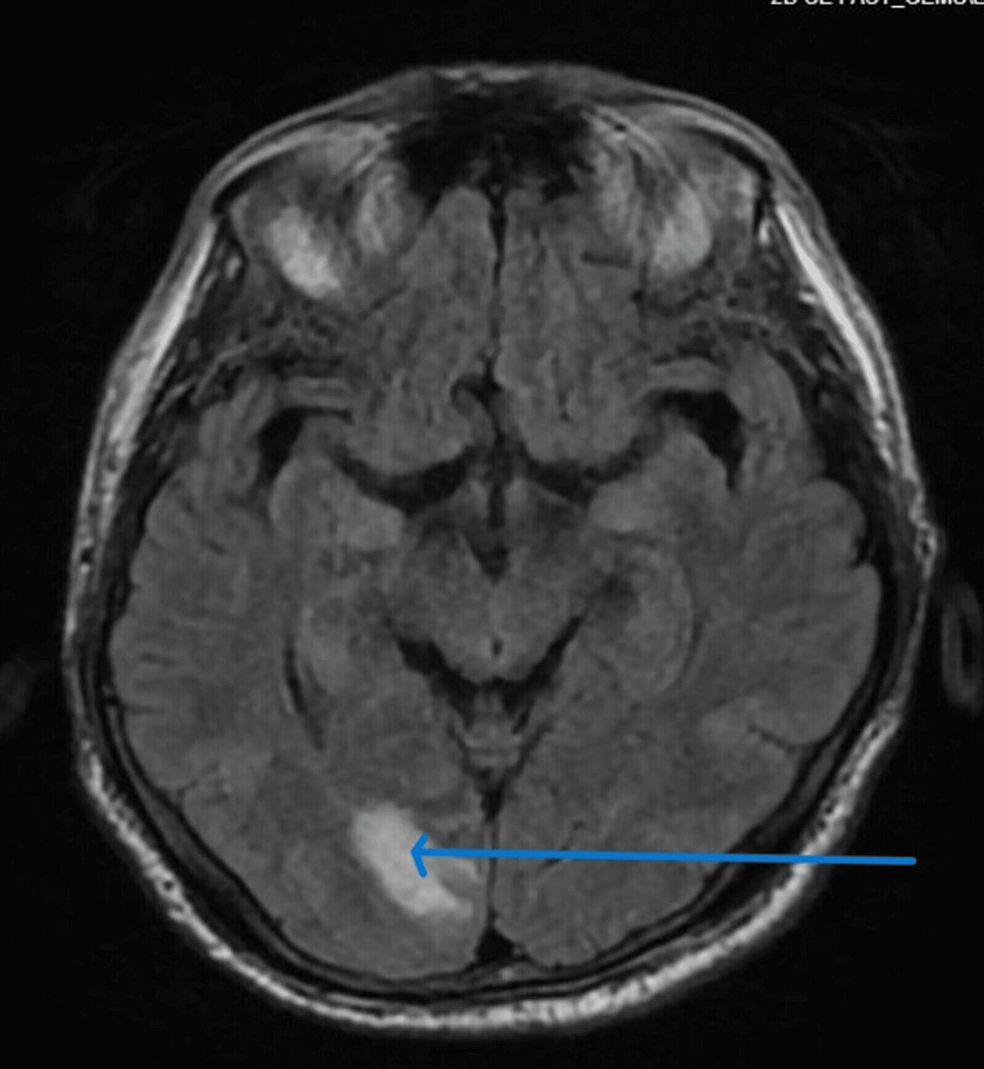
MRI of the brain demonstrating right occipital linear enhancement with surrounding vasogenic edema.
